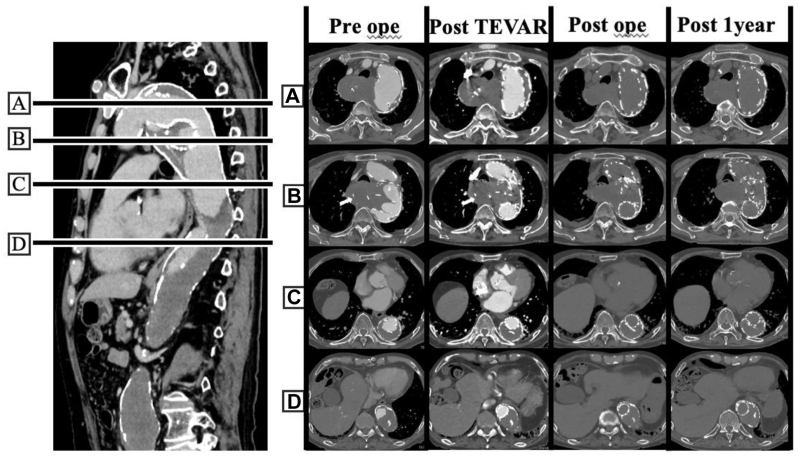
Preoperative oblique view along the aorta and serial axial computed tomography (CT) images at each time point. Preoperative oblique CT image along the aorta (*left*) and serial axial CT scans at four representative levels (A, distal arch; B, migrated stent graft; C, aortic valve; D, diaphragm) before surgery, after zone 1 thoracic endovascular aortic repair (TEVAR), after stent graft removal, and at 1-year follow-up. After zone 1 TEVAR, the blood flow within the aorta was completely excluded from the migrated stent graft without any endoleak. Postoperative CT scan, obtained without contrast because of concern for renal dysfunction, confirmed complete removal of the migrated stent graft. At 1 year, the pseudoaneurysm had neither enlarged nor regressed, indicating a stable condition.
